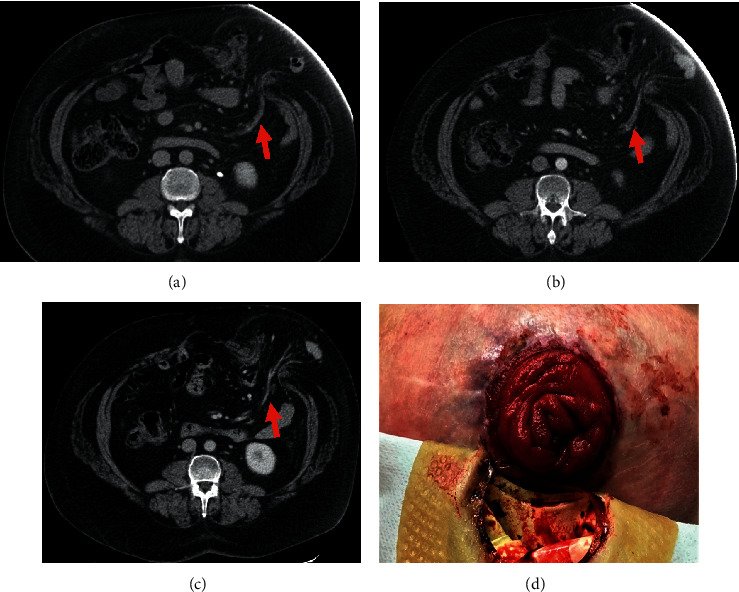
(a–c) Abdominal CT scans demonstrating dilated parastomal varices (red arrows). (d) Image depicting parastomal varices and hernia.
